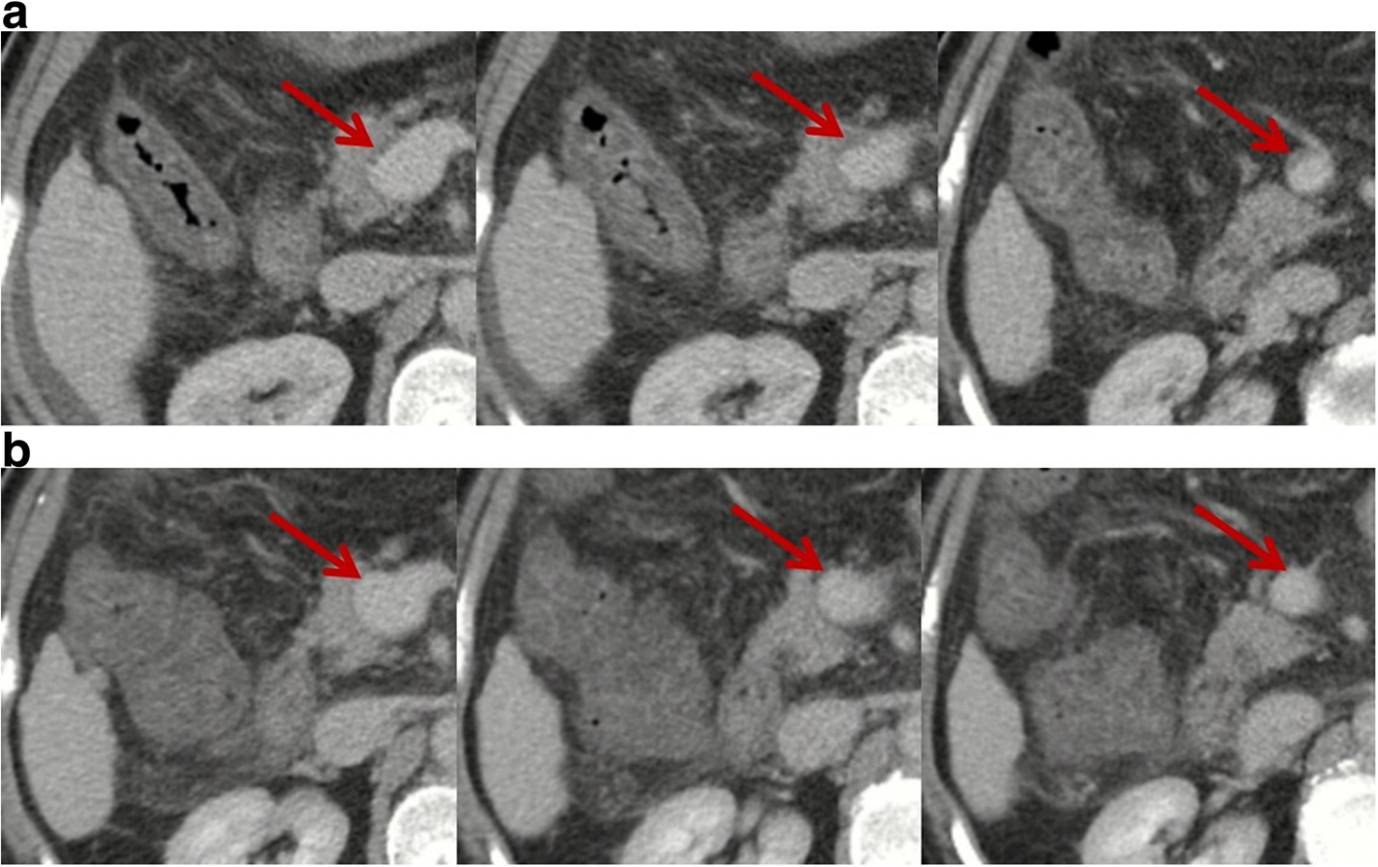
Contrast-enhanced computed tomography scans in a patient with transient PVT. Contrast-enhanced computed tomography performed in February 2017 demonstrated mild ascites, patent intrahepatic portal vein branches and splenic vein, mild thrombosis within main portal vein and superior mesenteric vein (SMV), and splenomegaly (panel a). *Red arrows* indicate mild thrombosis within the main portal vein and SMV. Notably, thrombus occupied less than 10% of the vessel lumen. In the absence of antithrombotic therapy, repeated contrast-enhanced computed tomography performed in April 2017 showed that intrahepatic portal vein branches, main portal vein, splenic vein, and SMV were patent (panel b). *Red arrows* indicate patent main portal vein and SMV
